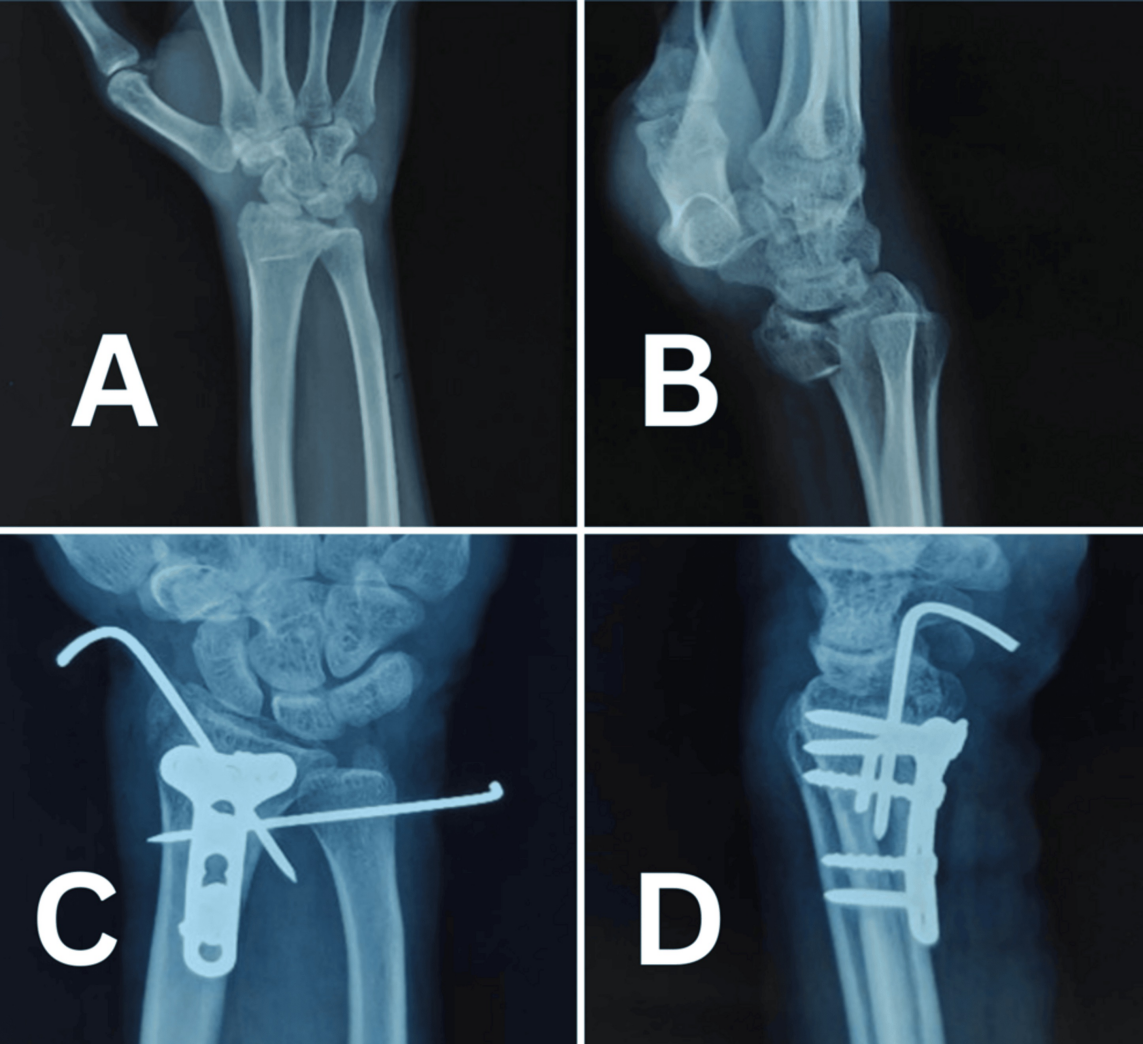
Case 3 wrist X-rays (A) Preoperative anteroposterior wrist X-ray showing a distal radius fracture. (B) Preoperative lateral wrist X-ray showing distal radius fracture. (C) One-month postoperative anteroposterior wrist X-ray showing fracture fixation and early union. (D) Postoperative anteroposterior wrist X-ray showing fracture fixation and union.
